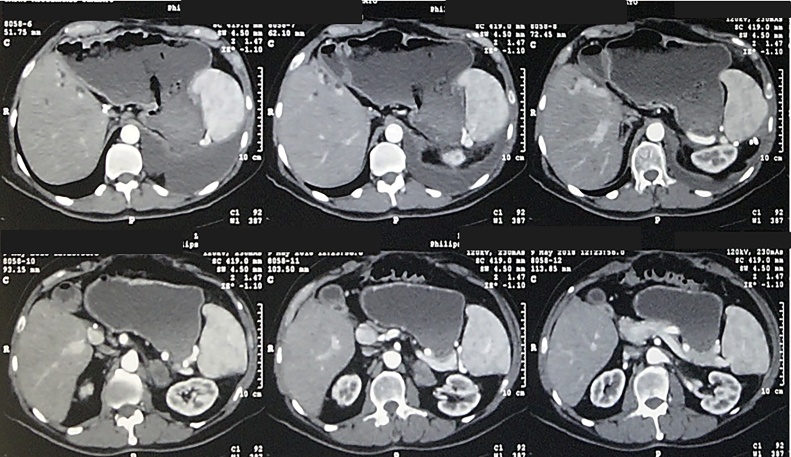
Abdominal computed tomography 6 month after excision of metastatic adrenal hepatocellular carcinoma.
